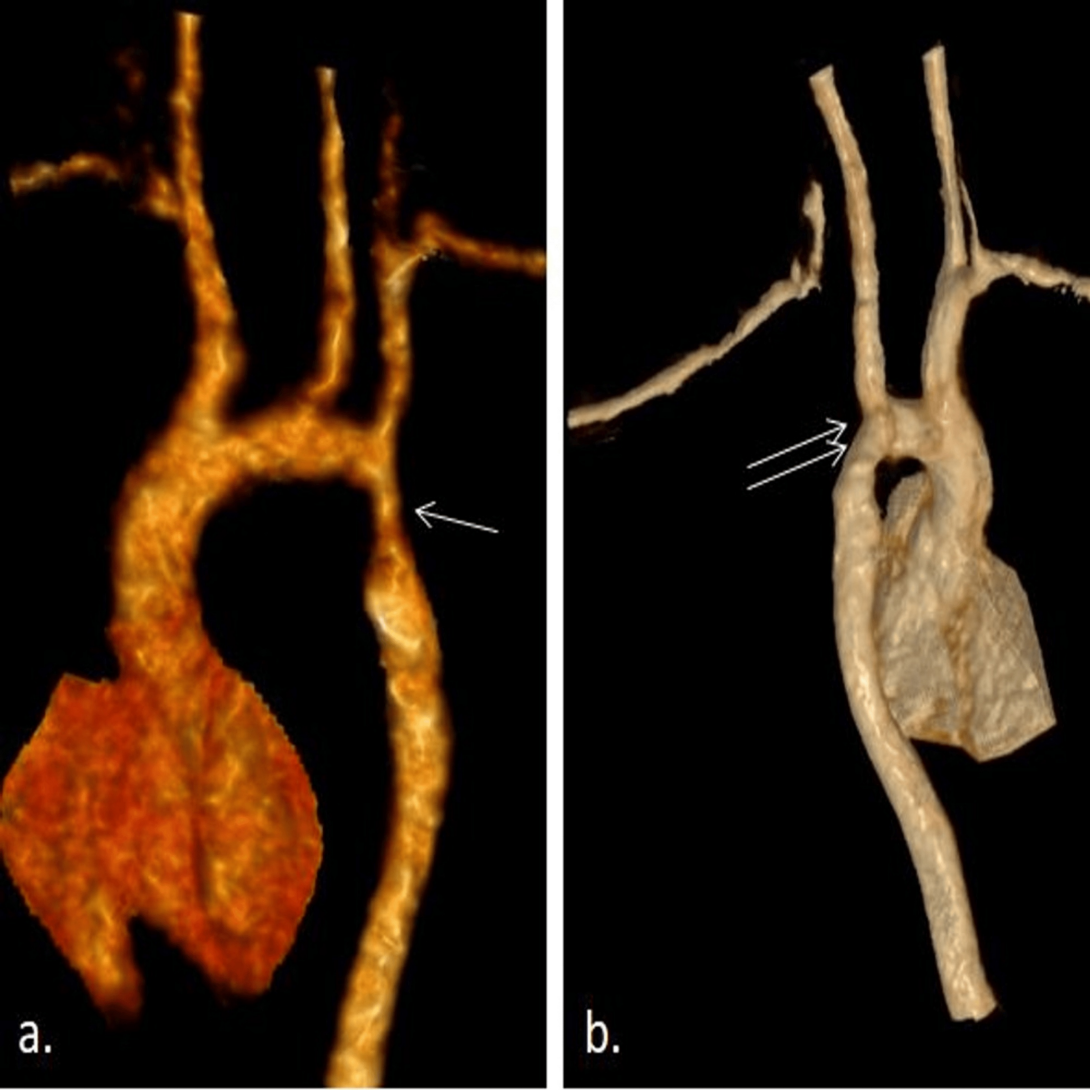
(a) Preoperative CT imaging showing the long-segment coarctation of the aorta (single arrow). (b) Postoperative CT imaging one year after the repair of coarctation of the aorta showing normal luminal continuity (double arrow) CT: computed tomography Image Credit: Authors (using BioRender and Keynote)
